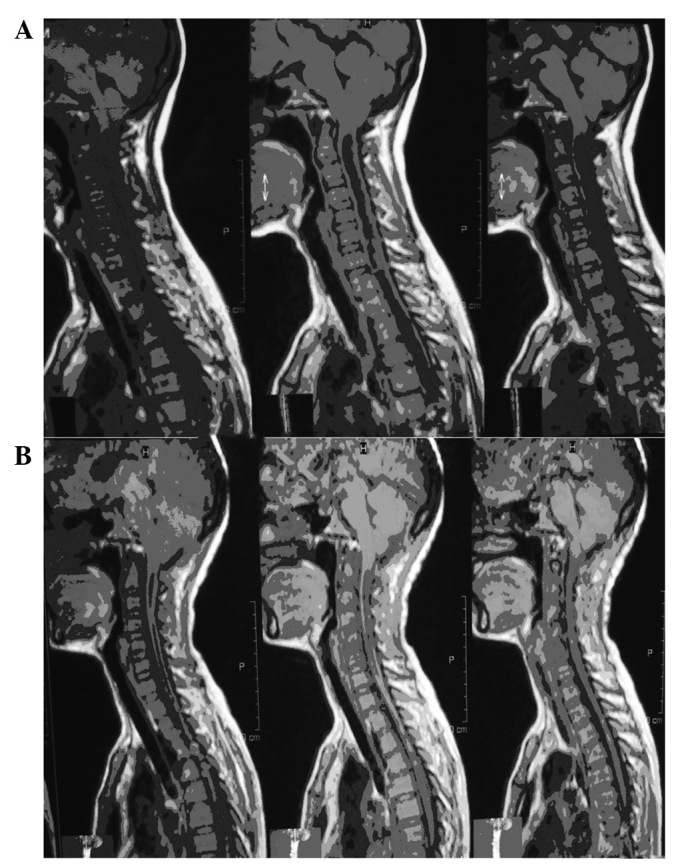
Preoperative and postoperative magnetic resonance images of patients with CMI complicated with SM. (A) Cerebellar tonsils have dropped below the foramen magnum to the atlantal level with severe SM prior to treatment. (B) Herniated cerebellar tonsils have moved upward to above the foramen magnum, the cisterna magna has effectively formed and the spinal syrinx has essentially disappeared 5 months after surgery. CMI, Chiari I malformation; SM, syringomyelia.
